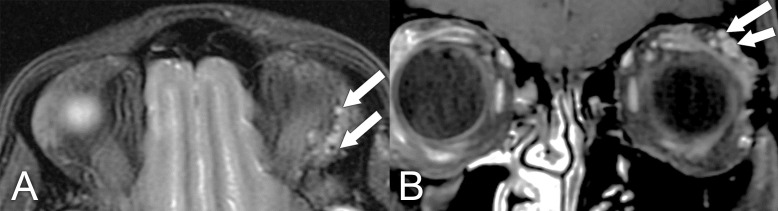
MRI in a 29-year-old woman who had undergone resection of a pleomorphic adenoma. More than 5 years later, she presented with some proptosis and multifocal recurrence in the surgical bed. (A) Axial T2 MRI with fat suppression shows multiple T2 hyperintense nodules in the superotemporal left orbit (arrows). (B) Coronal T1 postcontrast MRI with fat suppression in the same patient shows several enhancing nodules consistent with multifocal recurrent pleomorphic adenoma (arrows). The patient had surgical debulking of this multifocal lesion. The nodules were confirmed to be benign recurrent pleomorphic adenoma. It was not possible to remove all of these nodules, given their diffuse and extensive involvement of orbital soft tissue.
